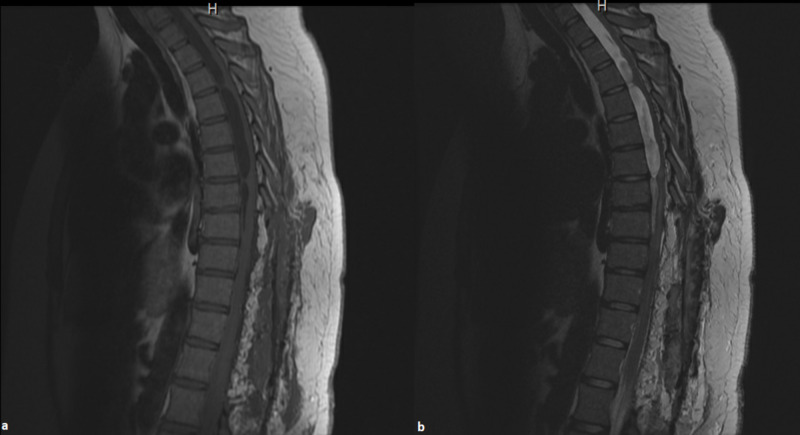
(a) T1 and (b) T2 sagittal thoracic MRI demonstrating postsurgical changes of laminectomy from T8 to L2. Arachnoid formations in the lower thoracic spine from T8 to T12 are no longer visualized.
